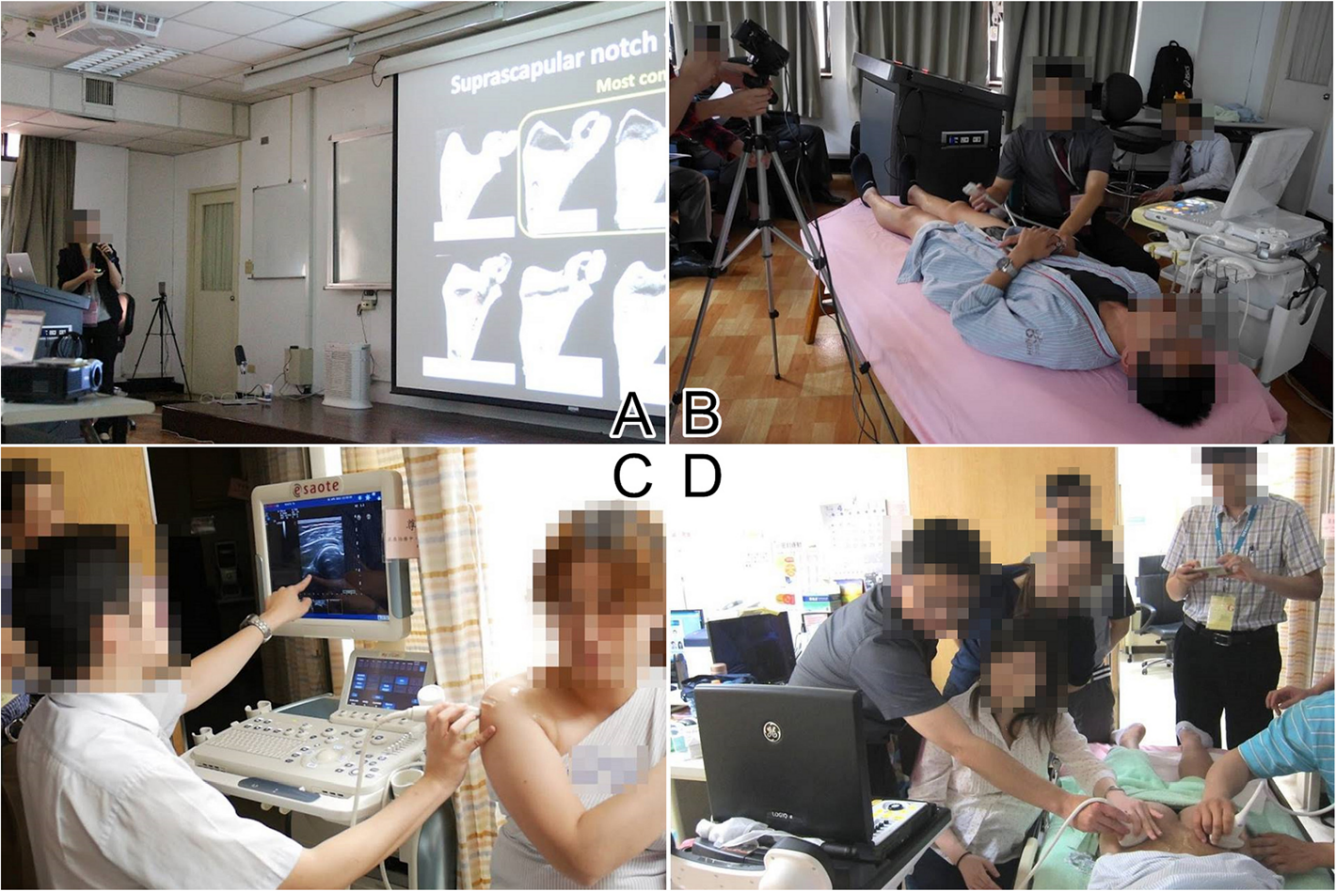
The course was divided into two parts. The didactic section consisted of (a) lectures based on six major joints and (b) standardization of scanning skills by using projections of ultrasound images and hand gestures on the screen in the auditorium room. The practical section comprised (c) demonstration of the sonoanatomy by the instructor and (d) scanning on the volunteer model by the attendee under the instructor’s assistance
